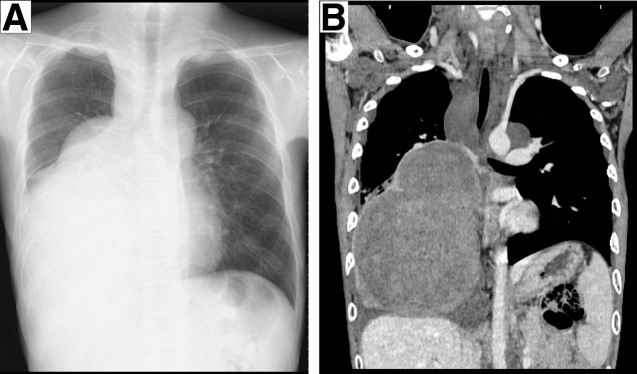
Imaging findings at the first visit. (A) The chest radiograph shows wide areas of consolidation in the right thoracic cavity. (B) Chest CT shows the development of a large tumor occupying the right middle and lower thoracic cavities, with atelectasis of the lower lobe of the right lung.
